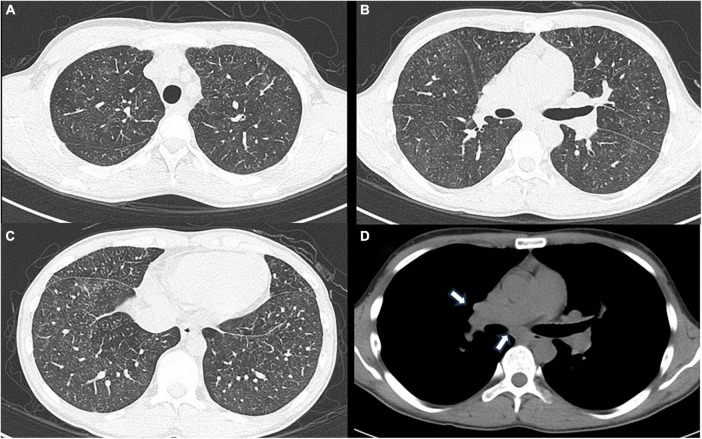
(A) Chest computed tomography using the lung window setting shows diffuse ground-glass shadows presumed to be fine nodules in the bilateral upper lobes, (B) middle and lingular lobes, (C) and bilateral lower lobes. (D) Chest computed tomography using the mediastinum window setting shows minor swelling of mediastinal lymph nodes, indicated by white arrows.
